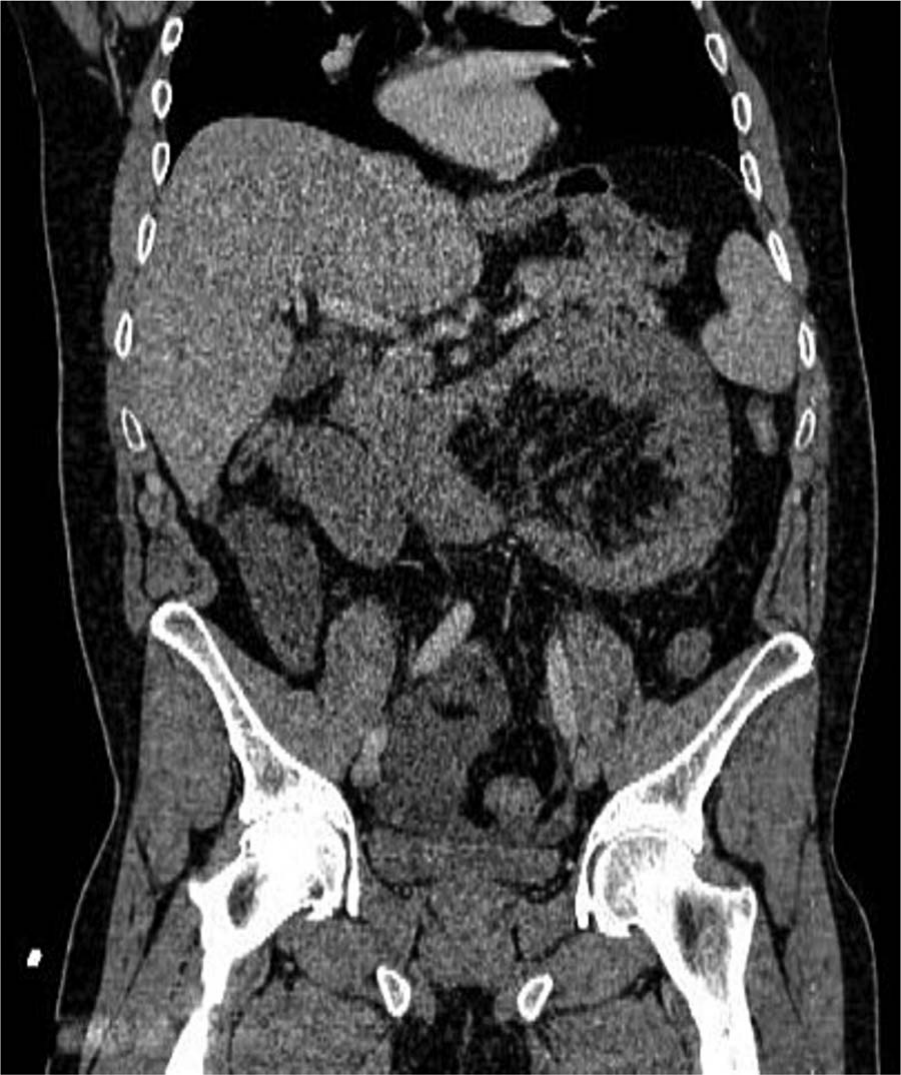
Coronal CT image showing clustered jejunal loops in the left abdomen with mass effect and convergence of mesenteric vessels toward the hernia orifice.
